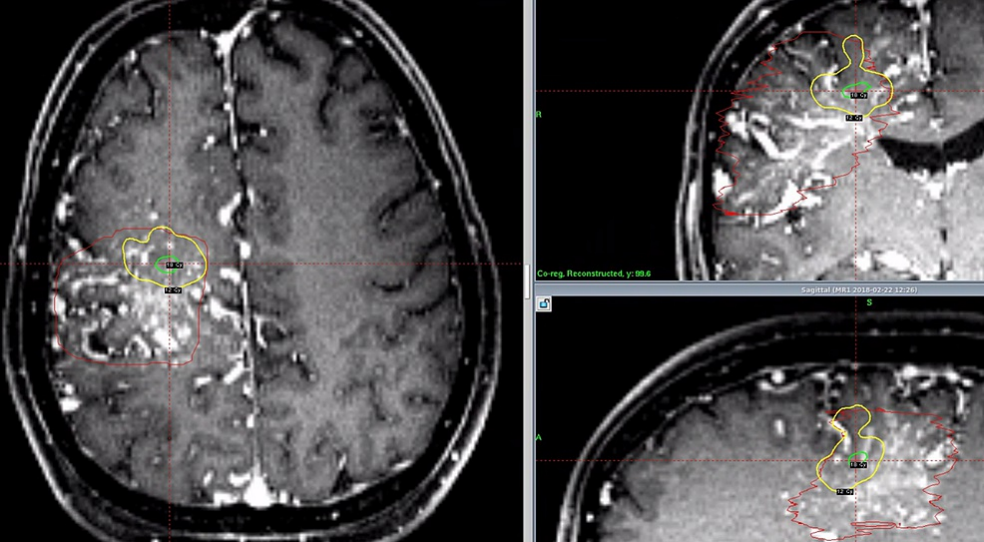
Axial (left), coronal (upper right), and sagittal (lower right) T1 post-contrast MRI of a large right-sided frontoparietal AVM treated with staged SRS. This AVM was divided into eight slices, and each received 12 Gy on separate days. MRI = magnetic resonance imaging; AVM = arteriovenous malformation; SRS = stereotactic radiosurgery
